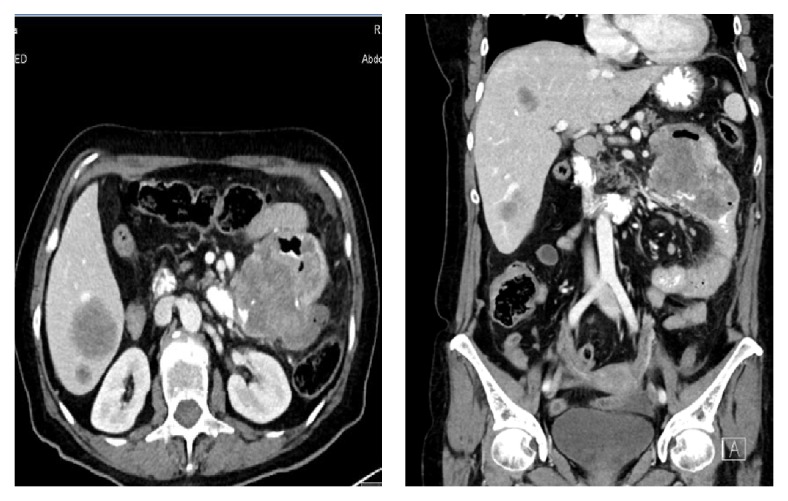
Axial and coronal CT scan showing a mass at proximal jejunum, with nearby free air and liver metastasis.
